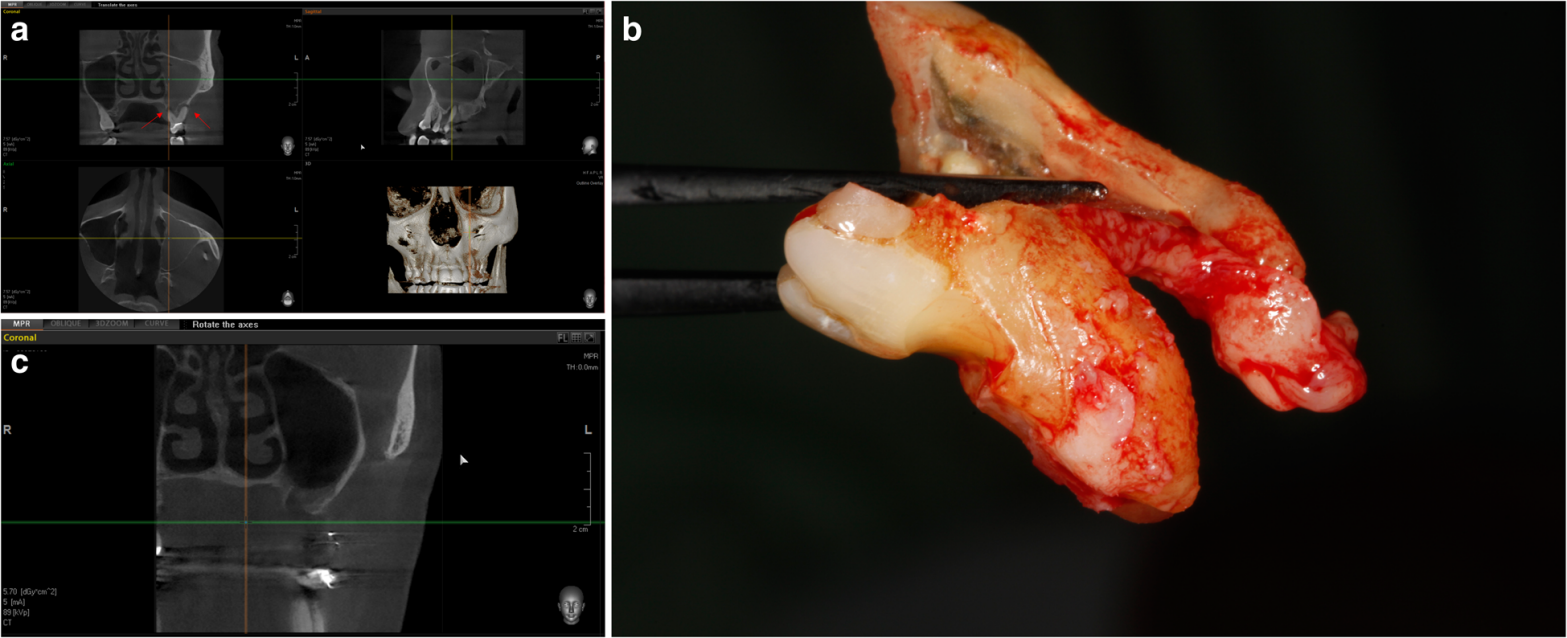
a CBCT of a molar with a periapical disease causing a maxillary sinusitis. b Extracted molar. c CBCT after a healing period of 3 months
